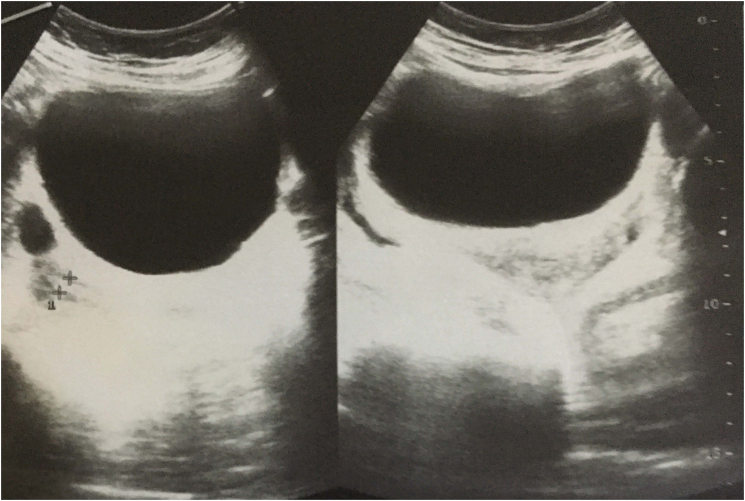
Ultrasonography showing distended gallbladder with thickening of gallbladder wall without any stone.
